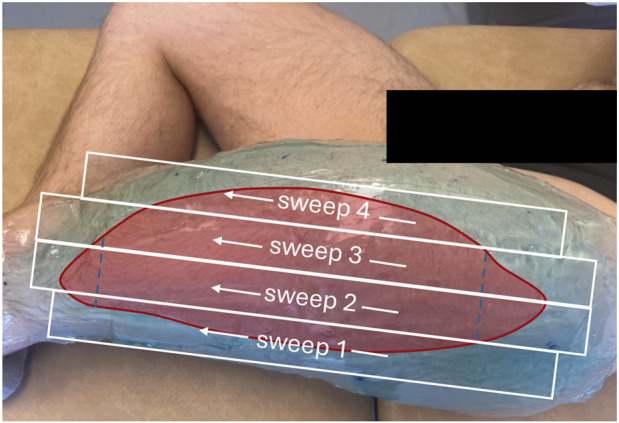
Participant positioned for ultrasound scanning with a coat of gel of about 1 cm. The red overlay represents the area of the vastus lateralis muscle. The white boxes denote the probe sweep trajectories and the dashed lines indicate the distal ends of the rectus femoris and tensor fascia latae, respectively.
